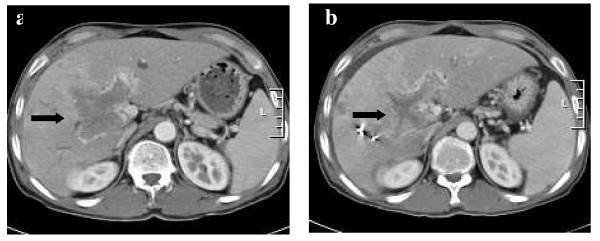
(a) The initial abdominal CT scan with the PVTT indicated by the arrow. (b) CT scan two months after SBRT and three courses course of TACE. This patients was classified as PR at 2 months after SBRT. (SBRT, stereotactic body radiation therapy).
